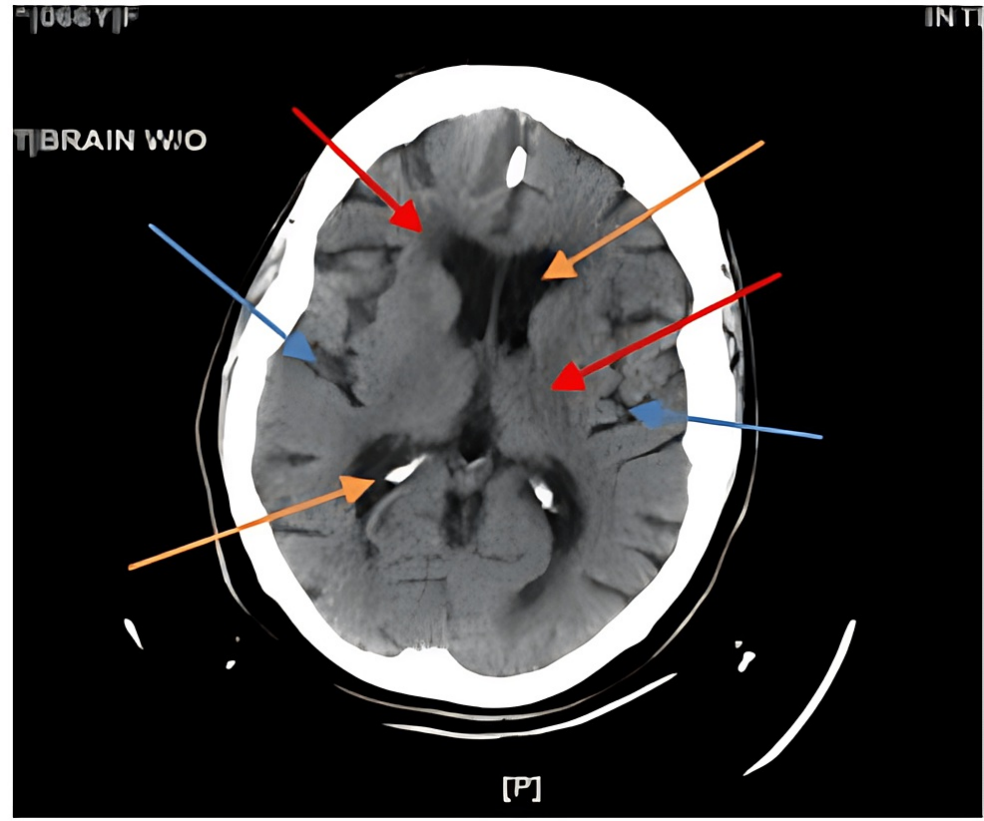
Axial CT without contrast scan of head done in 2022. Red arrows indicate areas of small vessel ischemia. Blue arrows indicate areas of volume loss. Orange arrows indicate enlarged ventricles.
